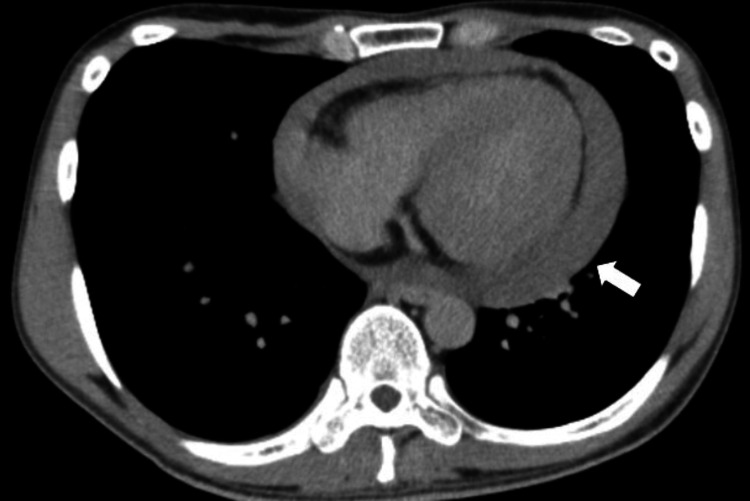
Chest CT (axial view) upon admission revealing significant pericardial effusion, due to increased pericardial fluid accumulation around the heart. The effusion is notable in the posterior region of the pericardium (arrow).
